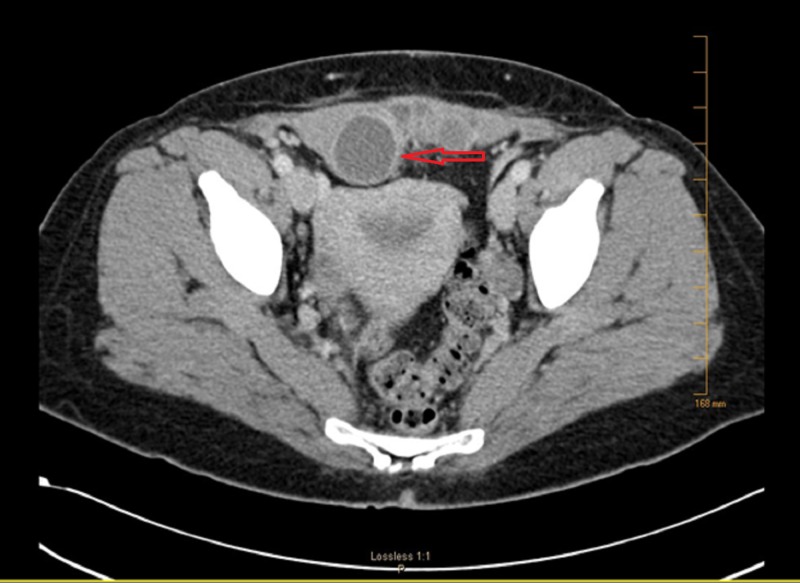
Axial computed tomography scan revealing a 6.2 x 6.8 x 3.6 cm complex cystic lesion involving the rectus muscle (arrow)
